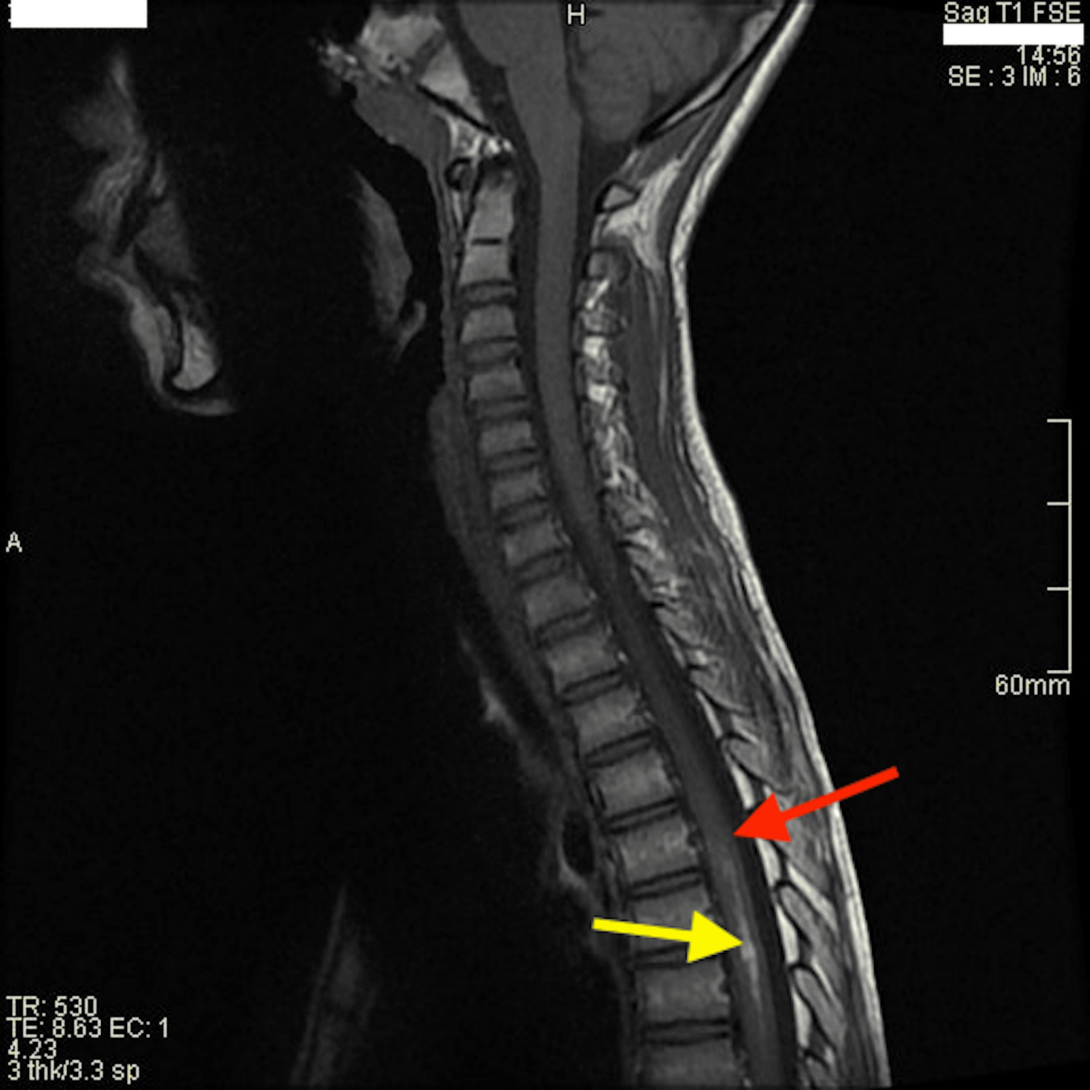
Underlying hemorrhage on T1-weighted imaging. Sagittal T1-weighted image with contrast product MRI demonstrating a non-enhancing isointense cavernoma (red arrow) and underlying hypersignal hemorrhage (yellow arrow).
